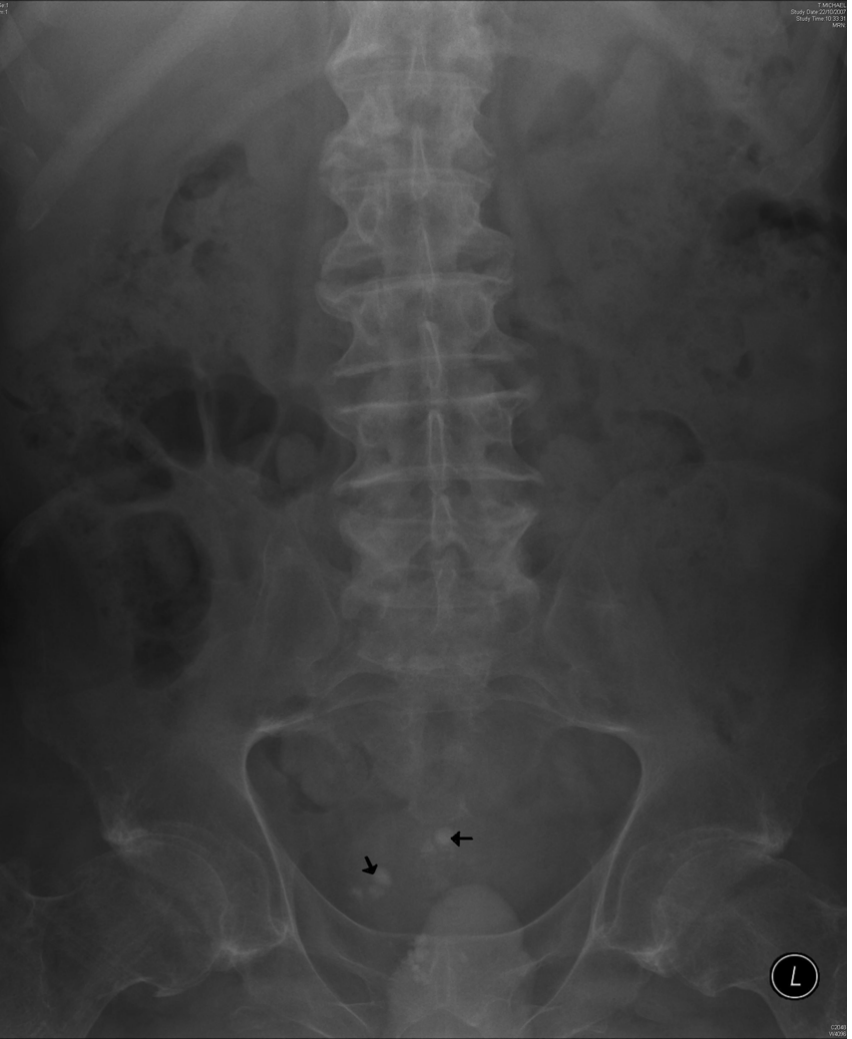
X-ray of abdomen taken on 22 October 2007 shows the presence of small stones in urinary bladder (arrow).
